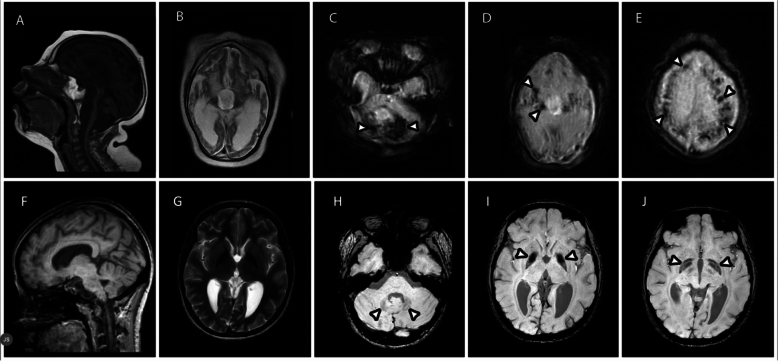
MRI images in a 4-year-old male with Aicardi-Goutieres syndrome (A–E) and an 18-year-old female with Down syndrome regression disorder (E–I). Sagittal T1-weighted (A, E), axial T2-weighted (B, F), and axial SWI (C–E, G–I) are shown. In AGS, there is diffuse cerebral and cerebellar atrophy with ex-vacuo ventriculomegaly (A, B), diffuse dystrophic calcification involving cerebellum (C), basal ganglia (D), and supratentorial cortex and white matter (E, arrowheads). In DSRD, there is mild cerebral atrophy (F, G). Dystrophic mineralization demonstrated in dentate nuclei of the cerebellum (H), basal ganglia (I, J, arrowheads)
